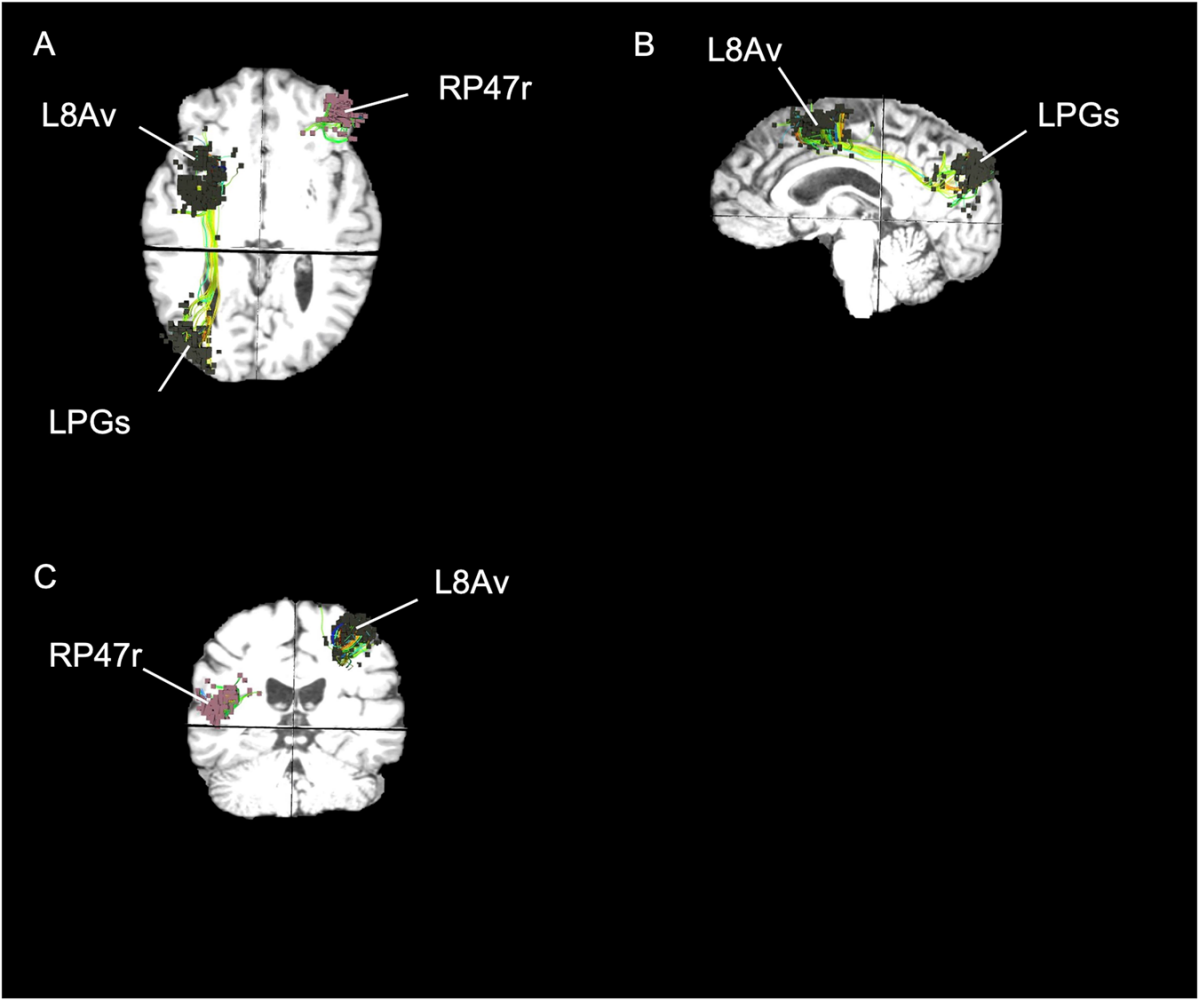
Anatomical locations of rTMS for Patient 1. (A) Axial, (B) sagittal, and (C) coronial visualization of rTMS targets superimposed on top of MRI of Patient 1. Patient 1 is a 48‐year‐old female with a diagnosis of MDD for 10 years. She had a baseline score of 8, posttreatment of 9, and follow‐up of 3 on the PSQI.
